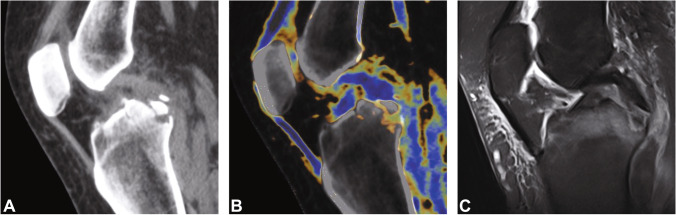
Standard unenhanced grayscale CT, color-coded collagen reconstructions, and unenhanced proton density-weighted MRI series with fat saturation of a 58-year-old patient show a tibial avulsion fracture of the posterior cruciate ligament (PCL) (A–C, arrows) following a dashboard injury. Color-coded collagen reconstructions and the MRI series demonstrate unambiguously a retained integrity of the thickened PCL (arrowheads), while there is some inhomogeneity of the PCL on standard grayscale CT series which led to a decreased readers’ diagnostic confidence when using protocol 1 compared to protocol 2 in this case. Abbreviations: PCL, posterior cruciate ligament
